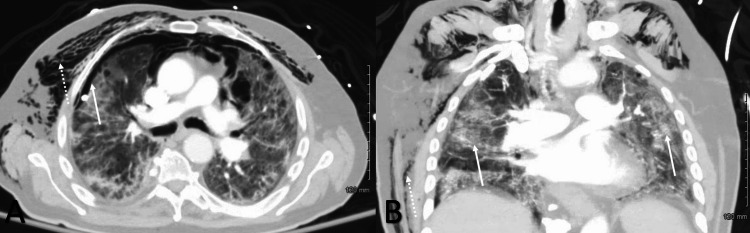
Computed tomography of the thorax with IV contrast A) Transverse computed tomography of the thorax with IV contrast showing soft tissue emphysema on the right and left (noted by the dashed white arrow), worse on the right along with residual small pneumothorax (noted by the solid white arrow). B). Coronal computed tomography atelectasis in bilateral lung spaces indicated with the solid white arrows and subcutaneous emphysema indicated with the dashed white arrow.
